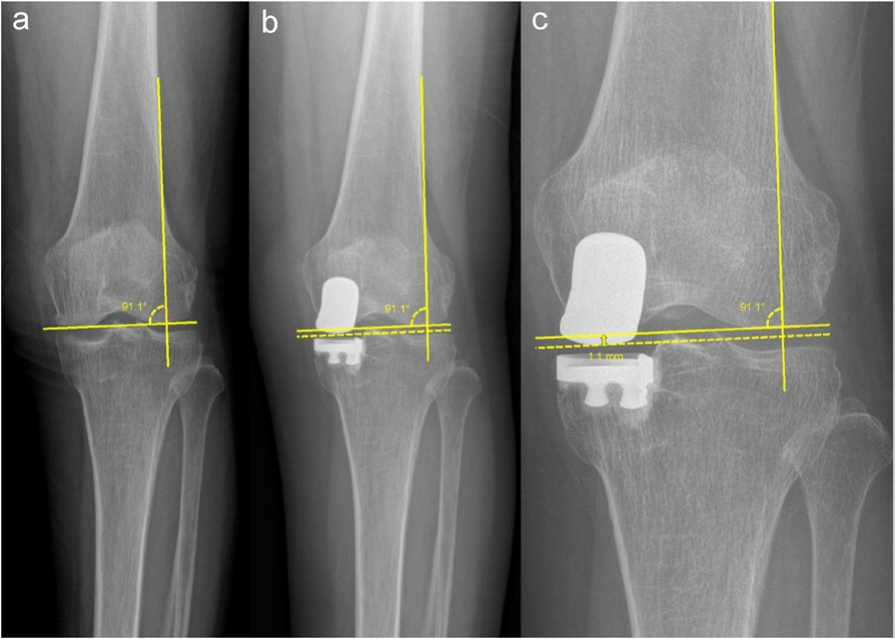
Methods of measurement of joint line height change. a On the preoperative radiograph, an angle is formed between a line tangential to the lateral femoral cortex and a line passing through the most distal points of the femoral condyles. b This reference angle is then applied to the postoperative radiograph, and the distance between the most distal point of the femoral component and the reproduced line is defined as the joint line height change, as described and validated by Herry et al. (c). In this patient, a joint line distalization of 1.1 mm is observed
